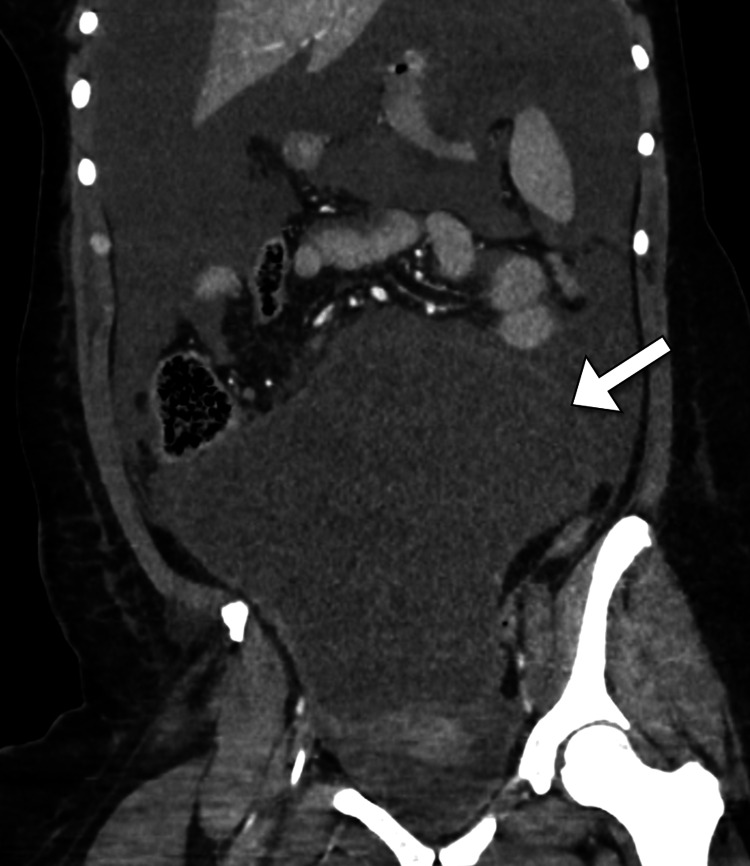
Coronal CT image of the abdominopelvic cavity shows a large cystic lesion (arrow) that is likely arising from the ovary with associated large intra-peritoneal free fluid. CT: computed tomography
